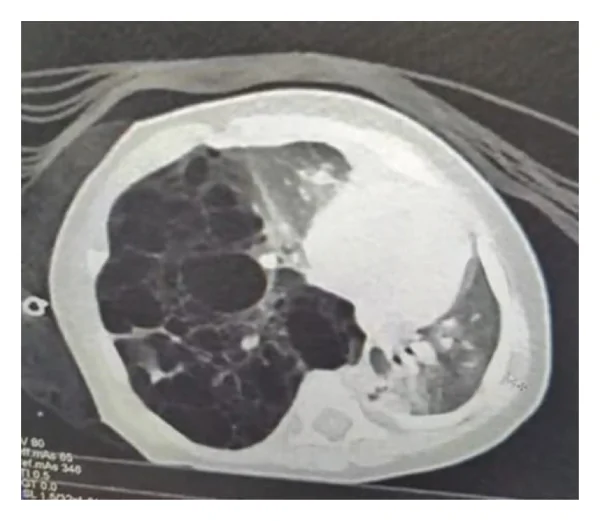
CT scan chest with contrast (axial view) shows regional hyperinflation on the right, composed of cysts of different sizes causing relaxation collapse of the anterior segment of the upper lobe with mediastinal shift to the contralateral side.
